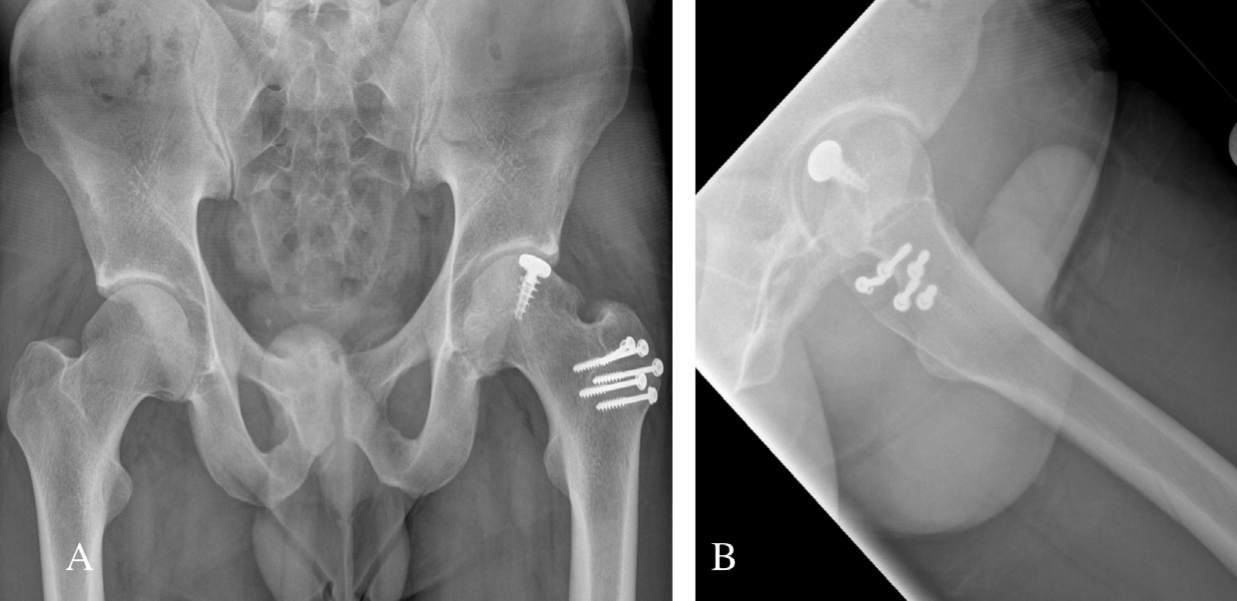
Patient 2: Final follow up X-ray at 5 years AP (A) and Lateral view (B).
